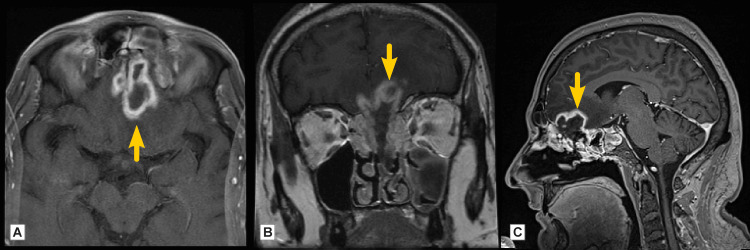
MRI post-contrast evaluation (Case 1) A: axial, B: coronal, and C: sagittal plane showing cerebral abscess (arrows) with perifocal edema and left-sided pansinusitis
